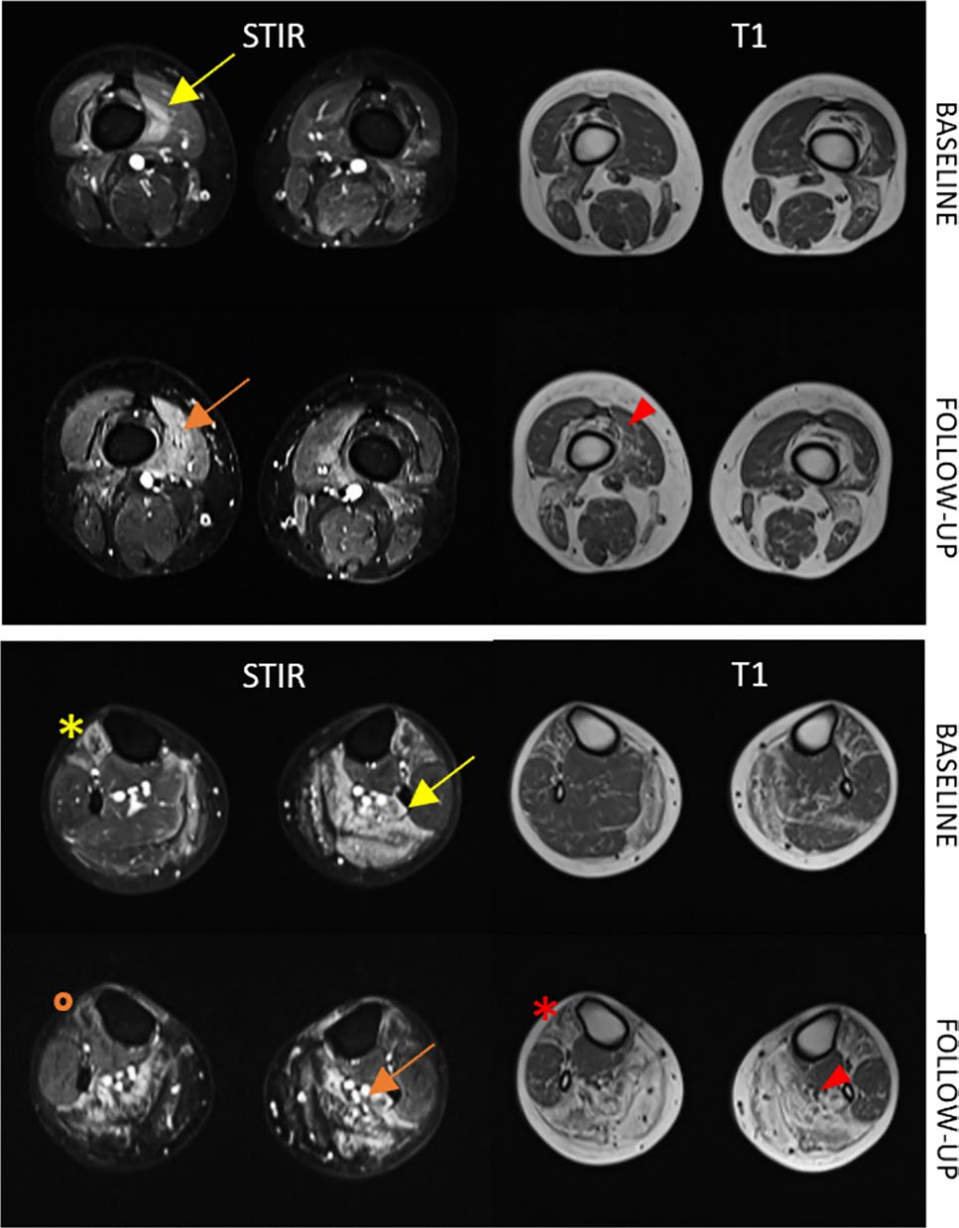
Muscle MRI findings. Axial plane of lower limbs in STIR and T1 sequences at BL and FU: STIR-positive right vastus medialis and left soleus at BL (yellow arrows) showed fat replacement progression in T1 sequences at FU study (red arrowheads) and still STIR-positive signal at FU (orange arrows); similarly STIR-positive right tibialis anterior at BL (yellow asterisks) showed T1 progression with complete fat replacement (red asterisks) but STIR negative (orange dot) at FU (P15, 36 years-old male at first MRI, FU duration 38 months, MIRS 3, E2 expansion class)
